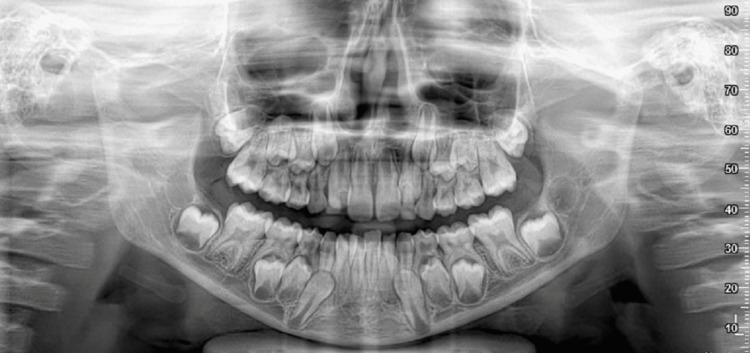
Follow-up orthopantomogram
